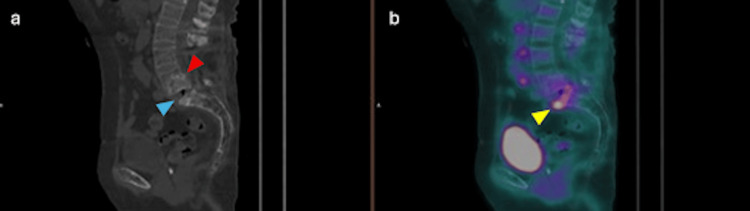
(a) Sagittal CT of the lumbosacral spine showing partial L4–L5 fusion (red arrowhead), and sclerotic/erosive changes at L5–S1 (blue arrowhead). (b) Sagittal 18F-FDG PET/CT showing intense hypermetabolism corresponding to the L5-S1 CT changes (yellow arrowhead), extending into the anterior soft tissues.
